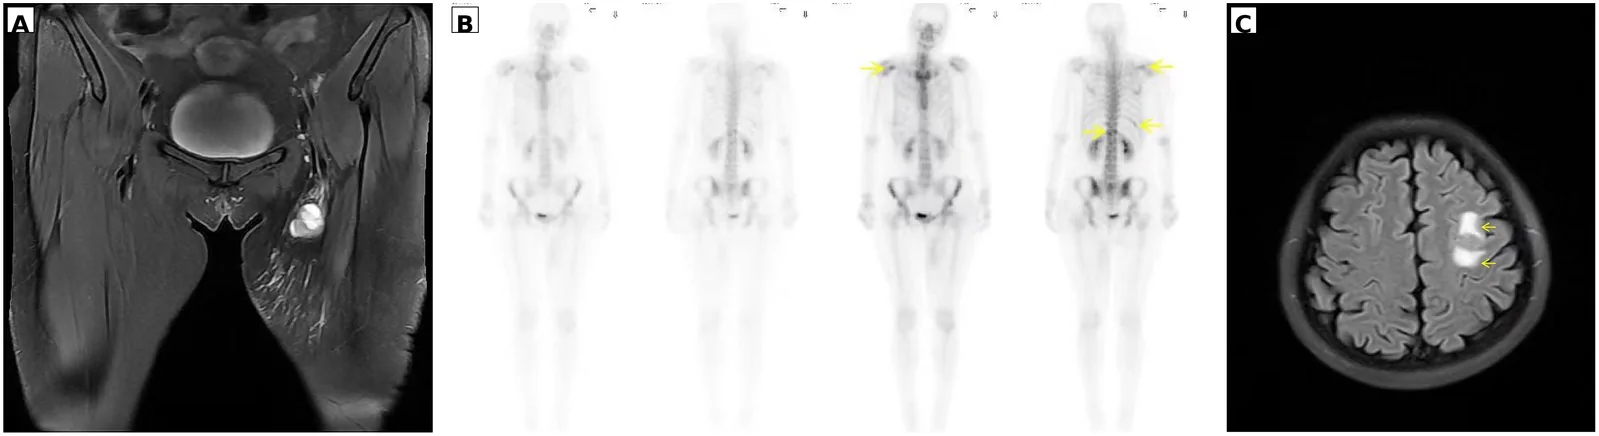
Imaging findings during disease progression. (A) Contrast-enhanced pelvic magnetic resonance imaging showing a heterogeneously enhancing cystic-solid mass in the left inguinal region. (B) Whole-body bone scintigraphy showing new radiotracer uptake in the right scapula, right posterior 10th rib, and T12 vertebra. (C) Contrast-enhanced brain magnetic resonance imaging showing the displayed left frontal metastatic lesion; a left cerebellar metastatic lesion was also reported radiologically but is not displayed in this panel.
